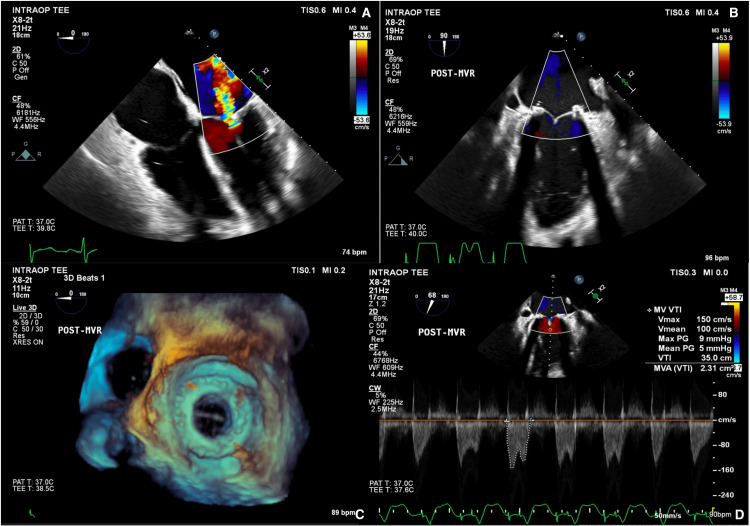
Intraoperative transesophageal echocardiography showing mitral valve anatomy before and after replacement and hemodynamics. A, B: intraoperative view of mitral valve before (A) and after (B) mitral valve replacement (MVR). C, D: 3D echocardiogram of the new 31 mm Mitris (Edwards Lifesciences Corporation, CA, USA) valve in the mitral position (C) and 2D echocardiogram and new valve with measurements.
